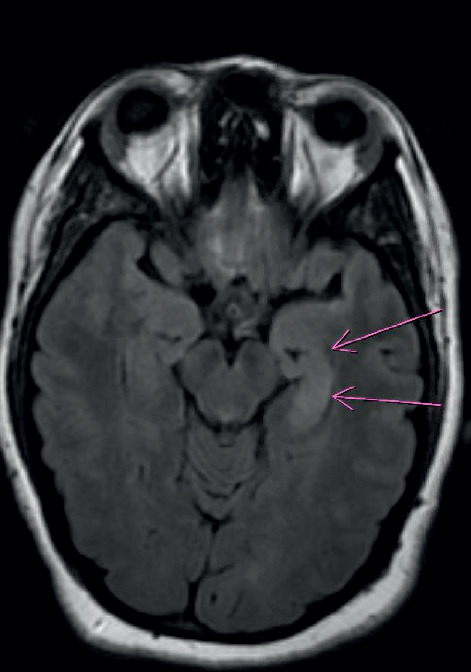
Brain MRI showing T2 flair hyperintensity in the medial temporal lobes with left lobe (pink arrows) revealing greater hyperintensity than right lobe.
